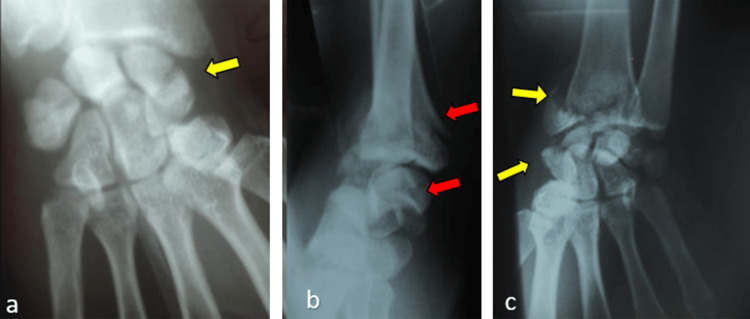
(a) and (c) anteroposterior (AP) radiographs of the wrist showing scaphoid and distal radius fractures (yellow arrows) and (b) profil radiograph showing a trans-scapho retrolunar fracture with a distal radius articular fracture (red arrows).
